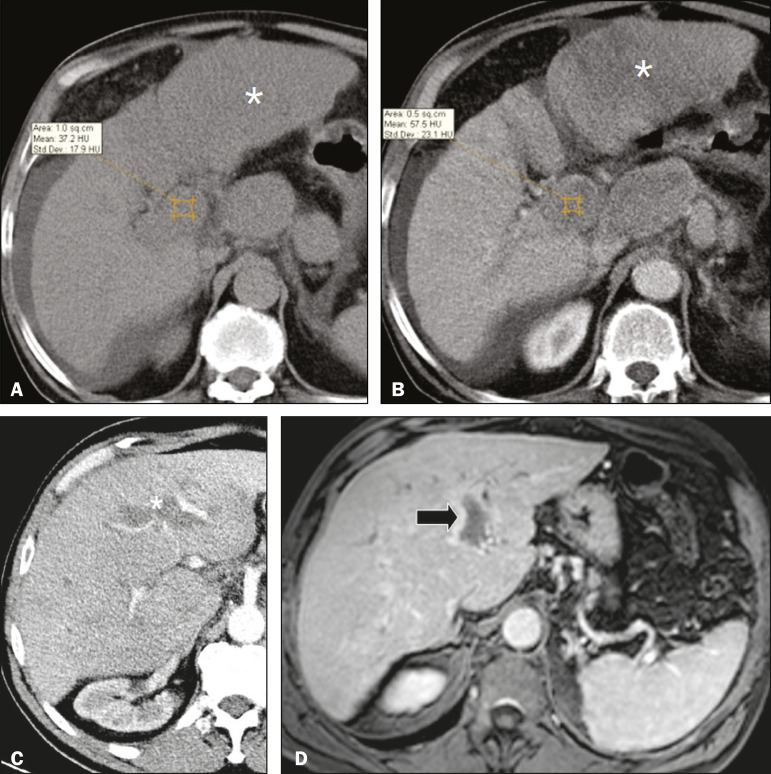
A,B: CT scan showing a tumor thrombus secondary to HCC (asterisk). Axial sections acquired before and after contrast administration (A and B, respectively), showing 20 UH of enhancement in the thrombus (i.e., 37 UH in the pre-contrast phase and 57 UH in the post-contrast phase). In addition, there is expansion of the vessel, also related to the tumor component. C: Axial CT scan of a patient with a vascular thrombus, showing no enhancement of the thrombus in the portal phase (asterisk). D: Contrast-enhanced T1-weighted MRI scan of a patient with a vascular thrombus in the left portal vein branch (black arrow), showing no enhancement.
